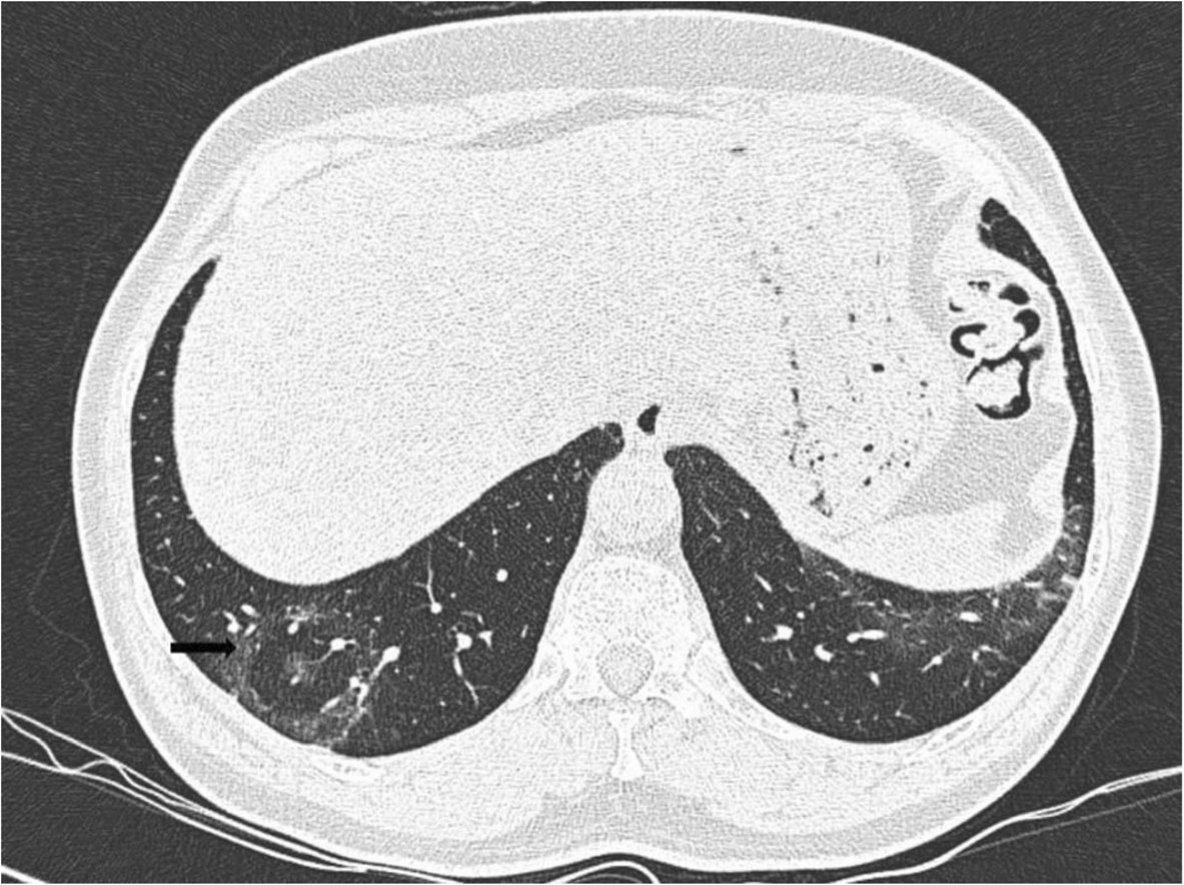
Bull’s eye sign. Axial CT thorax of a 55-year-old COVID pneumonia patient showing central ground glass opacity surrounded by an inner ring of air and an outer ring of ground glass in the right lower lobe
